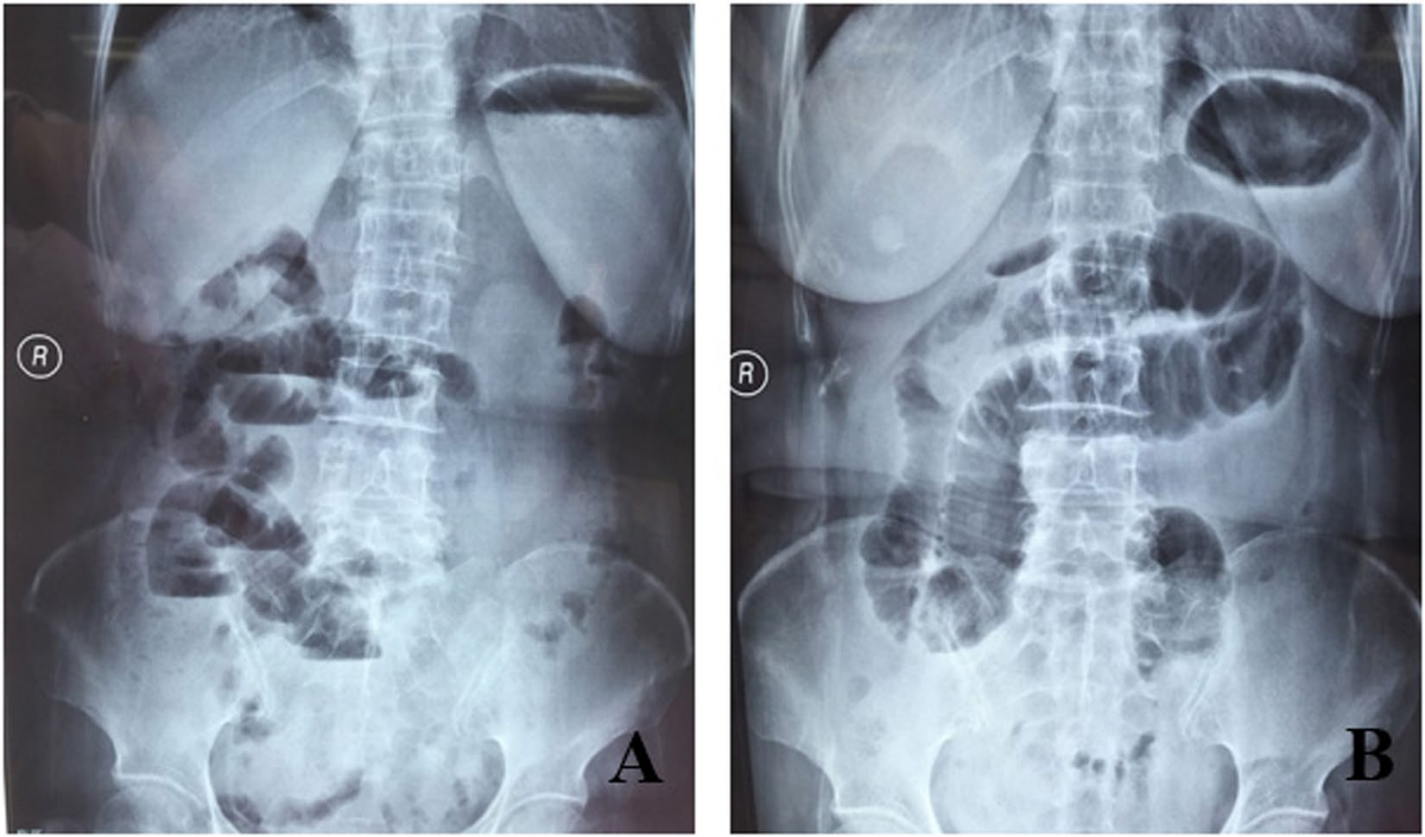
Two representative abdominal X-ray in 2 patients with intestinal. obstruction, but confirmed with IH by surgery. (A) one patient with multiple abdominal surgical history had abdominal pain and abdominal distension. Abdominal X-ray diagnosed with intestinal obstruction, surgery confirmed with IH diagnosis. (B) one patient had abdominal distension and vomiting at 4 days after radical resection of sigmoid colon cancer. Abdominal X-ray diagnosed with intestinal obstruction, surgery confirmed with IH diagnosis.
